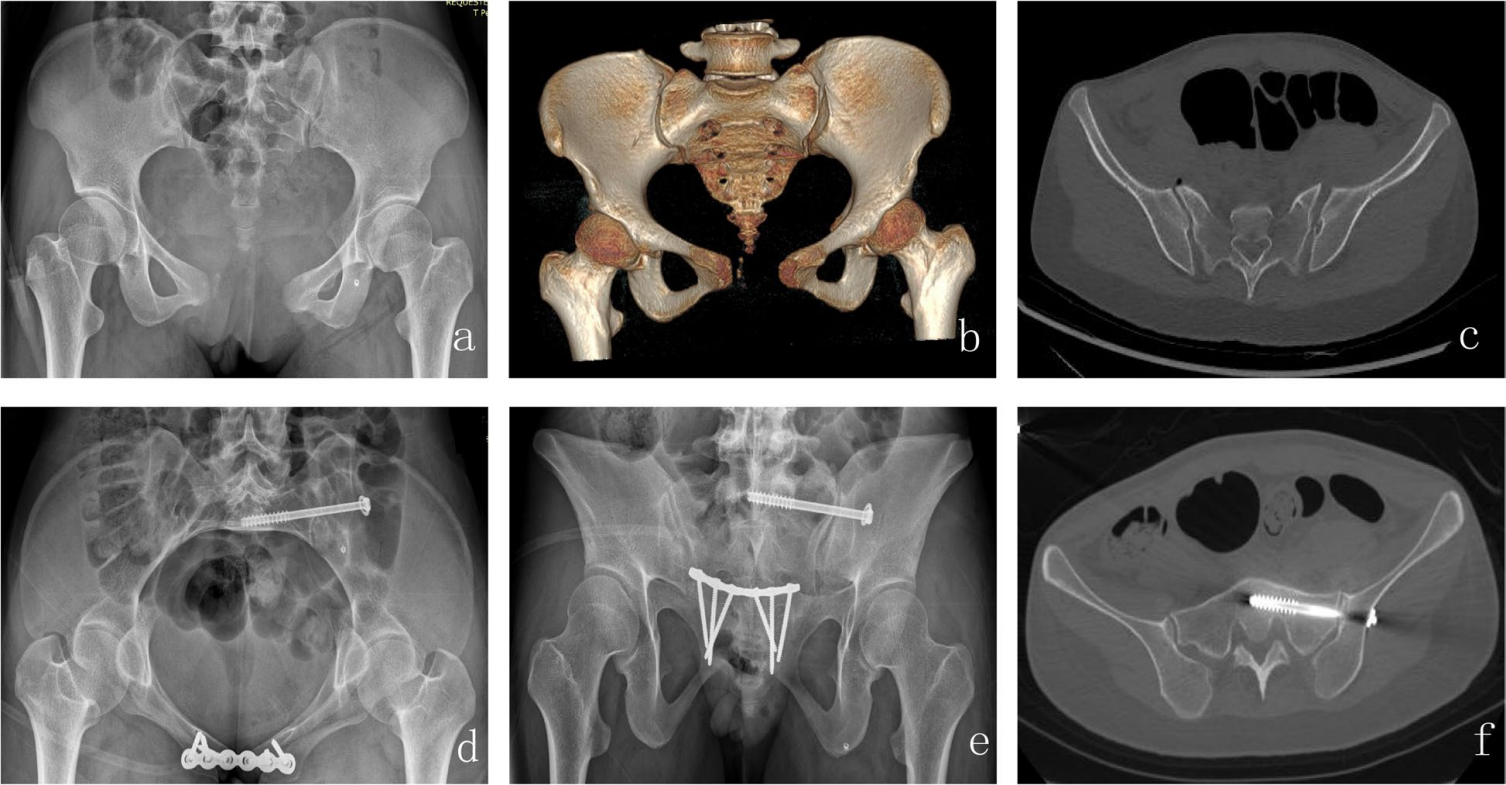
This is a 23-year-old woman who was injured in a traffic accident. The fracture was identified as Tile B1 on the preoperative X-ray. Using conventional X-ray fluoroscopy, the posterior ring was secured using iliosacral screws. a Preoperative anterior–posterior view of pelvis; b 3D image of pelvic CT; c Preoperative CT scan showing left sacroiliac joint separation; d Postoperative inlet view; e Postoperative outlet view; f Postoperative axial CT image
